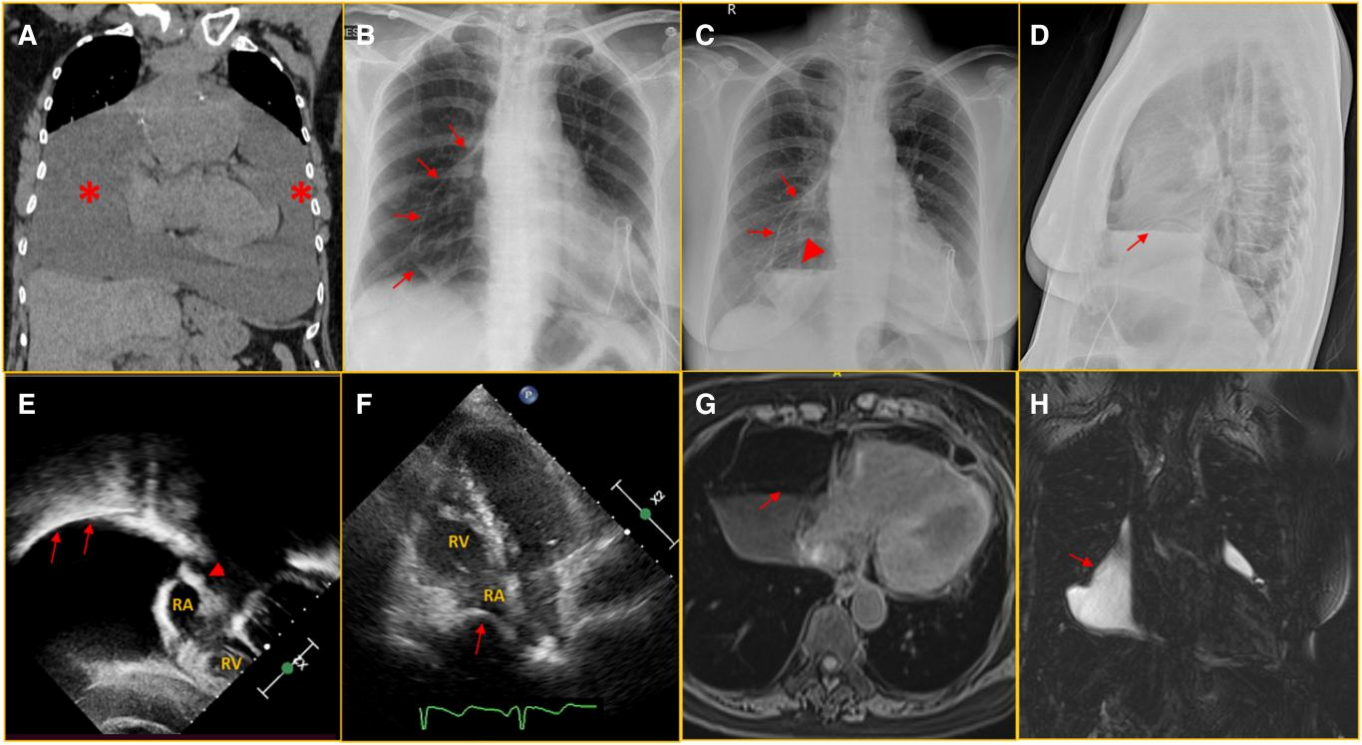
(*A*) Cardiac computed tomogram on coronal section showing massive pericardial effusion (asterisk—pericardial effusion). (*B*) Chest X-ray in anteroposterior view showing pneumopericardium (arrows—pericardium). (*C* and *D*) Chest X-ray in anteroposterior and lateral view (*D*) showing pneumopericardium (air–fluid level—arrowhead; arrows—pericardium). (*E*) 2D echocardiogram in modified subcostal four-chamber view showing pericardial effusion with air–fluid level (arrows) (arrowhead—interatrial septum). (*F*) 2D echocardiogram in apical four-chamber view showing pericardial effusion with diastolic right atrium collapse (arrow). (*G* and *H*) Cardiac magnetic resonance imaging in axial post-contrast T_1_ fat saturation (*G*) and coronal heavily T_2_-weighted (*H*) section confirming chylopneumopericardium (arrows—air–fluid level).
